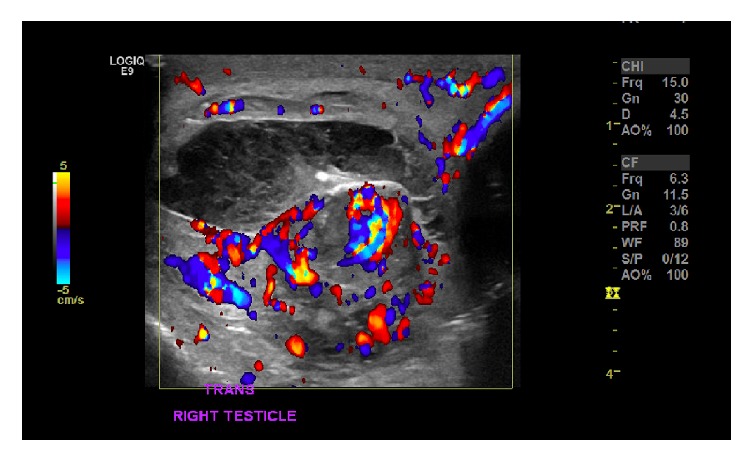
Doppler ultrasound on postoperative day one of the right testicle demonstrating robust return of vascular flow to the testicle following necrotic epididymis and hematoma excision.
